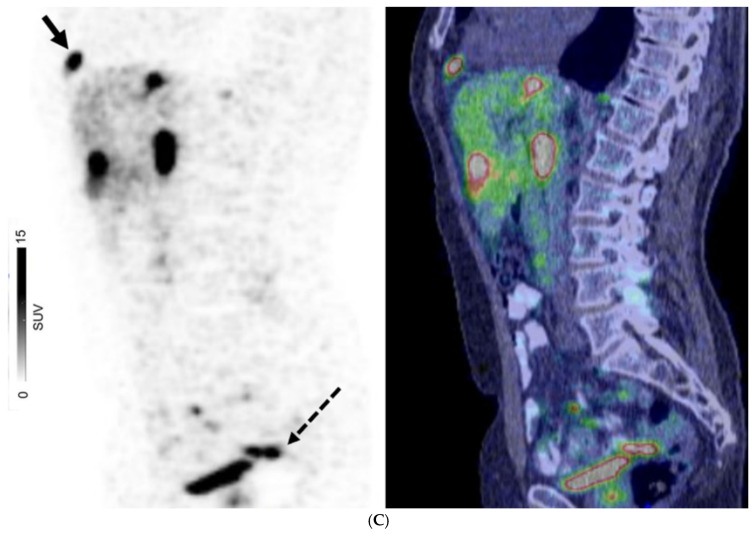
A 67-year-old man with well-differentiated G1 small bowel NET, Ki 67 = 3% metastatic to liver. Staging CT showed mid-ileal tumor, regional nodal metastases, and bilobar liver metastases [CT stage: n1M1a]. ^68^Ga-DOTATATE PET/CT (A,B) Axial and Coronal—CT (left), fused PET/CT (middle) and PET (right); (C) Sagittal—Fused PET/CT (left) and PET (right) shows in addition, extrahepatic metastases including extraregional nodes including supraclavicular and cardiophrenic nodes (arrows in (A,C)), and peritoneal metastases (dotted arrows in (B,C)); overall, PET stage: n1M1c.
